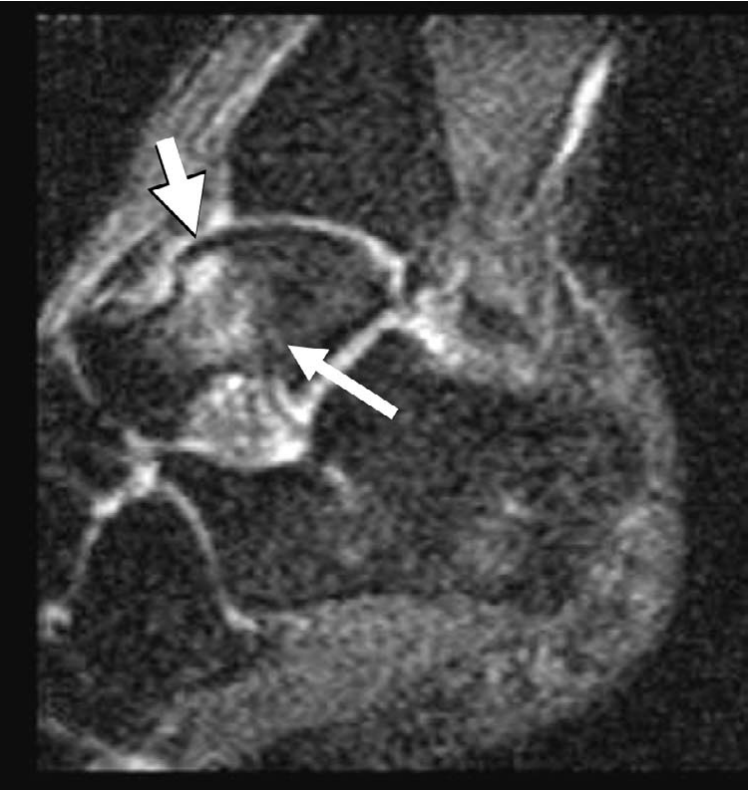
Sagittal STIR MR image of a 25-year-old male shows patchy edema signal within the body of the talus (long arrow), which extends to the subchondral region of the talar dome (short arrow). This same edema pattern was observed in nine of the twelve ankles and may be related to chronic repetitive stress.
